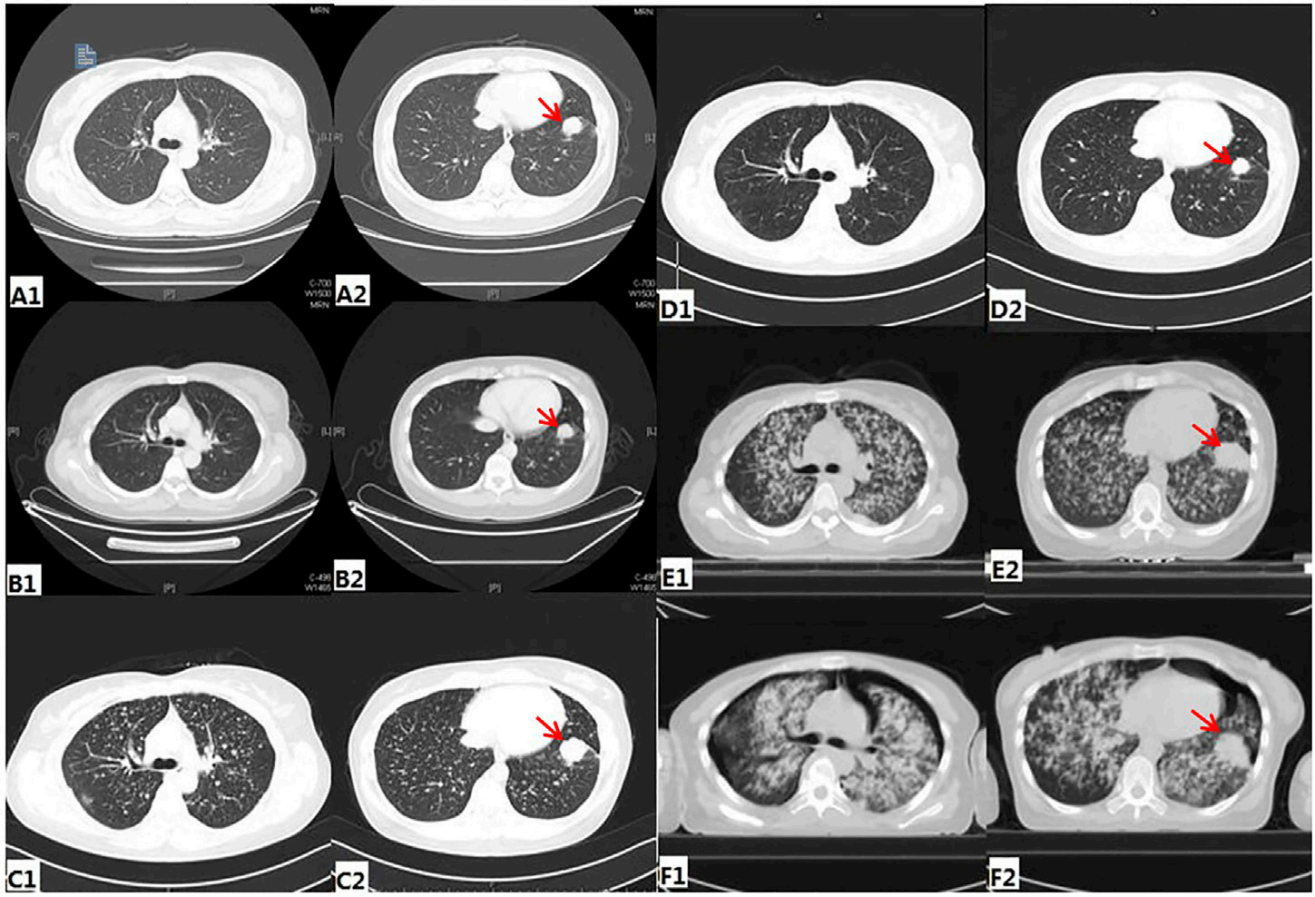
Radiologic findings during treatment (A) before any treatment (B) Post-treatment image after three cycles of pemetrexed and cisplatin (C) before Afatinib treatment (D) CT of the chest showing PR after 1month of Afatinib (E) before nivolumab treatment (F) CT of the chest showing PD after 4 cycles of Nivolumab.
